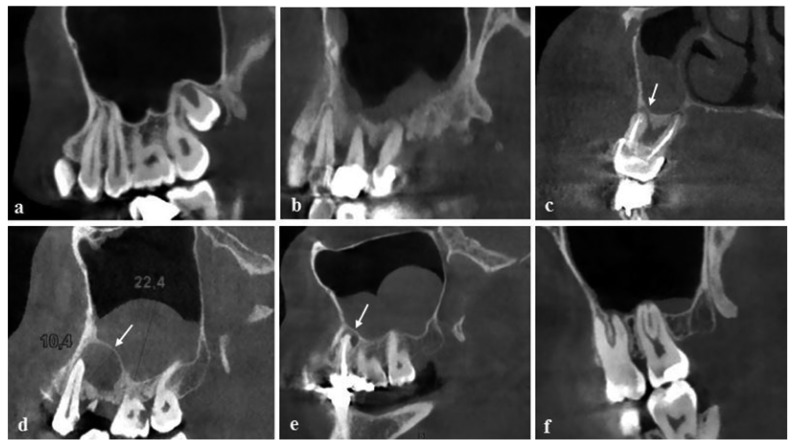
a-f.(a) Normal mucosa of the maxillary sinus, (b) severe periodontitis with exacerbating features and mucosal thickness, (c) mucosal thickness due to severe periodontal bone loss and apical periodontitis related with maxillary right first molar with root canal therapy , (d) mucosal thickness associated with the lesion in contact with the sinus floor, (e) tooth with root canal therapy and apical periodontitis with a well-defined radiolucent area and mucosal thickness, (f) sinus mucosal thickness due to root apex entered the sinus floor. Arrows indicate the periapical lesion.
